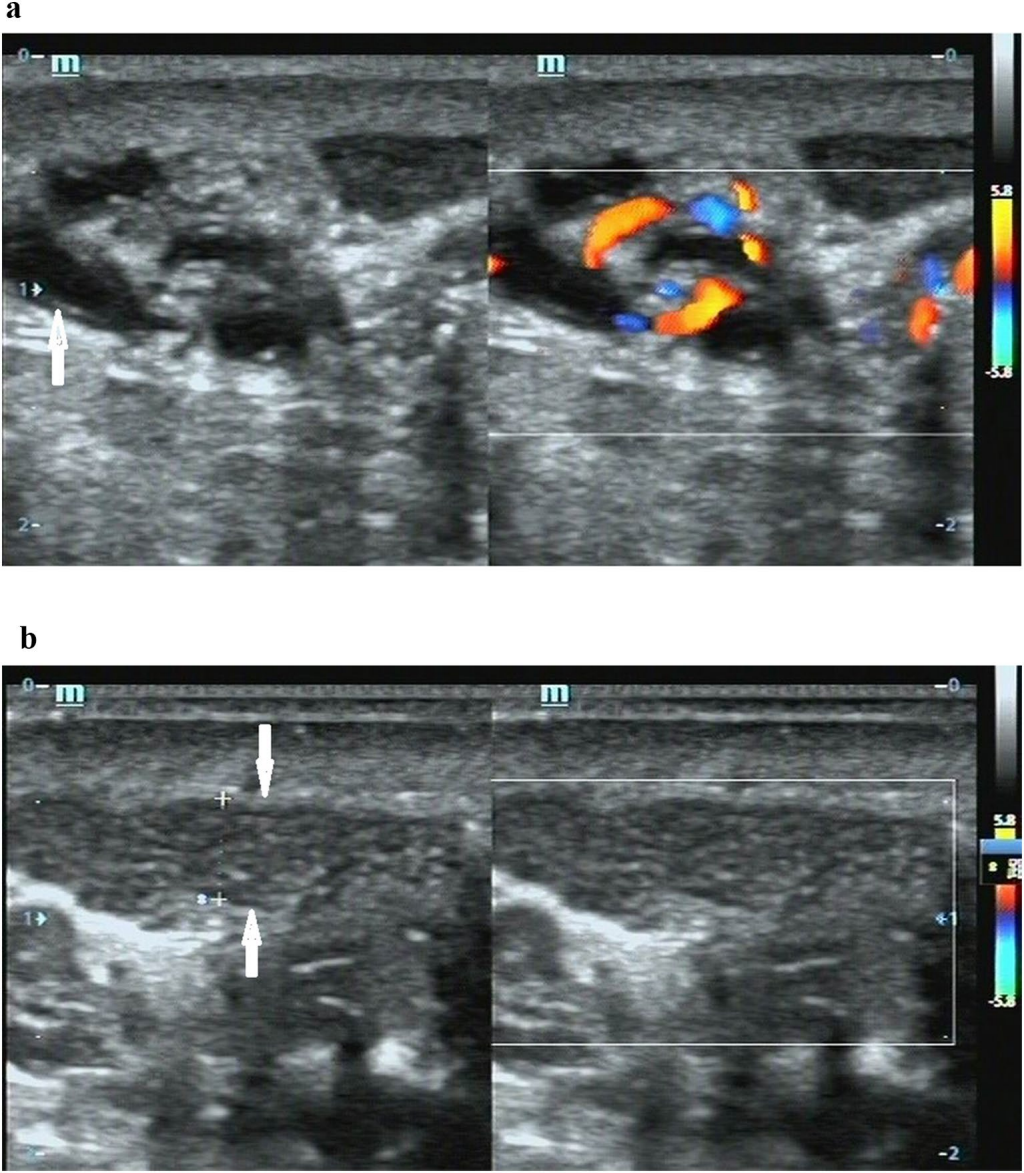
A 37-year-old man with dilated proximal vas deferens. The dilated proximal vas deferens present fluid-filled anechoic tubular structures without color Doppler flow signal in the region of the supratesticular region on duplex ultrasound at standing position with Valsalva maneuver, and the wall of the tubular structures is thicker than that of the pampiniform vein (arrow, a); the left epididymis present multiple thin tubular structures without color Doppler flow signal (arrows, b).
